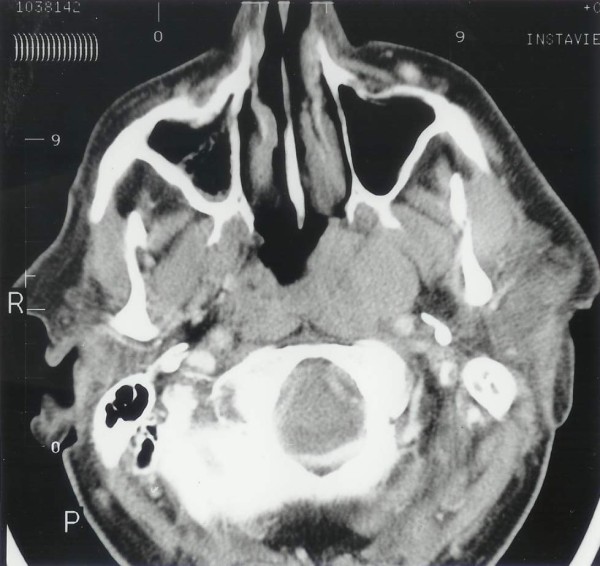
Computed tomography scan showing a well circumscribed uniform density mass on the left side of the posterior wall of the nasopharynx.
